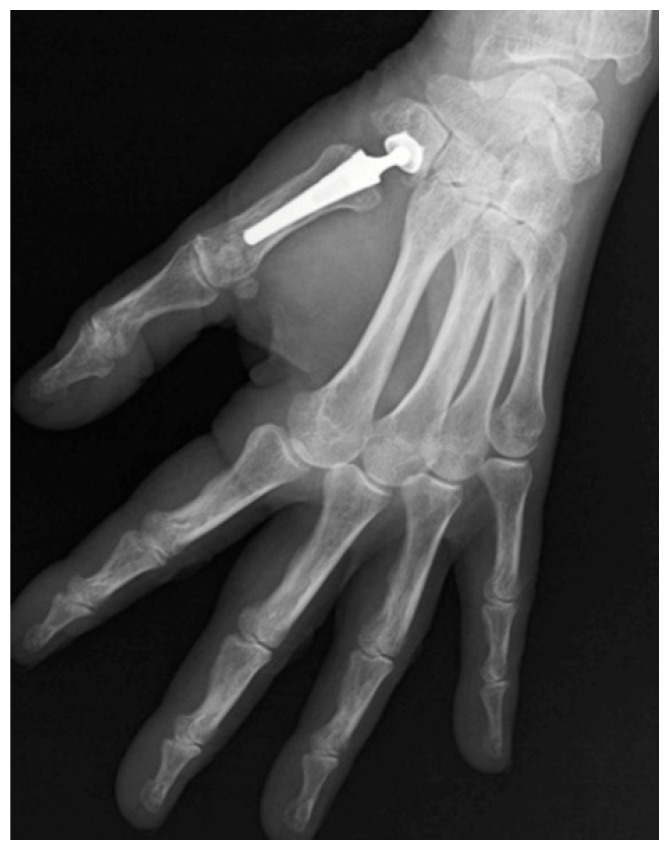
Radiographic image showing trapeziometacarpal joint prosthetic replacement.
